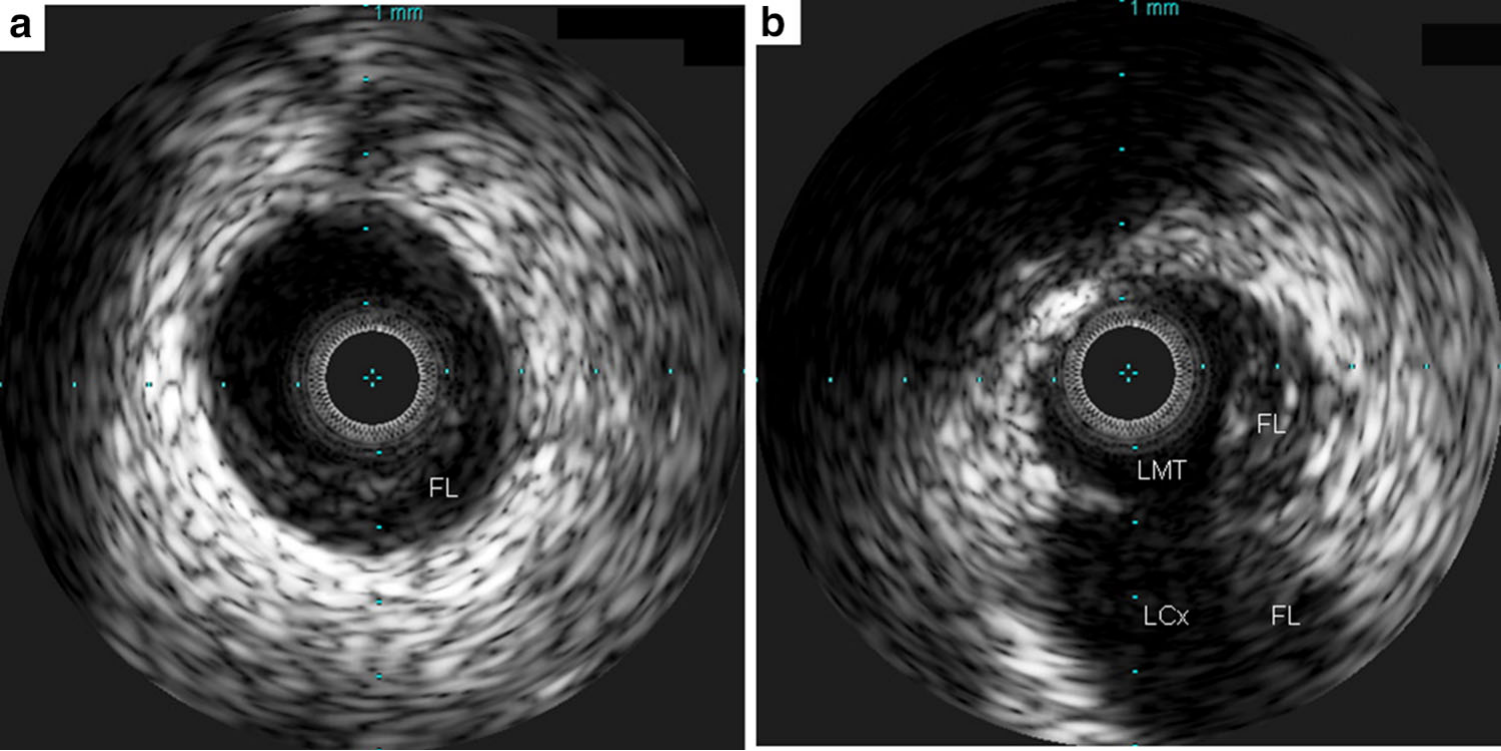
Intravascular ultrasound of the left coronary artery. a Left anterior descending artery showing the false lumen. b Left main trunk: the false lumen and the dissection extending to the left circumflex artery. *FL* false lumen, *LCx* left circumflex artery, *LMT* left main trunk
